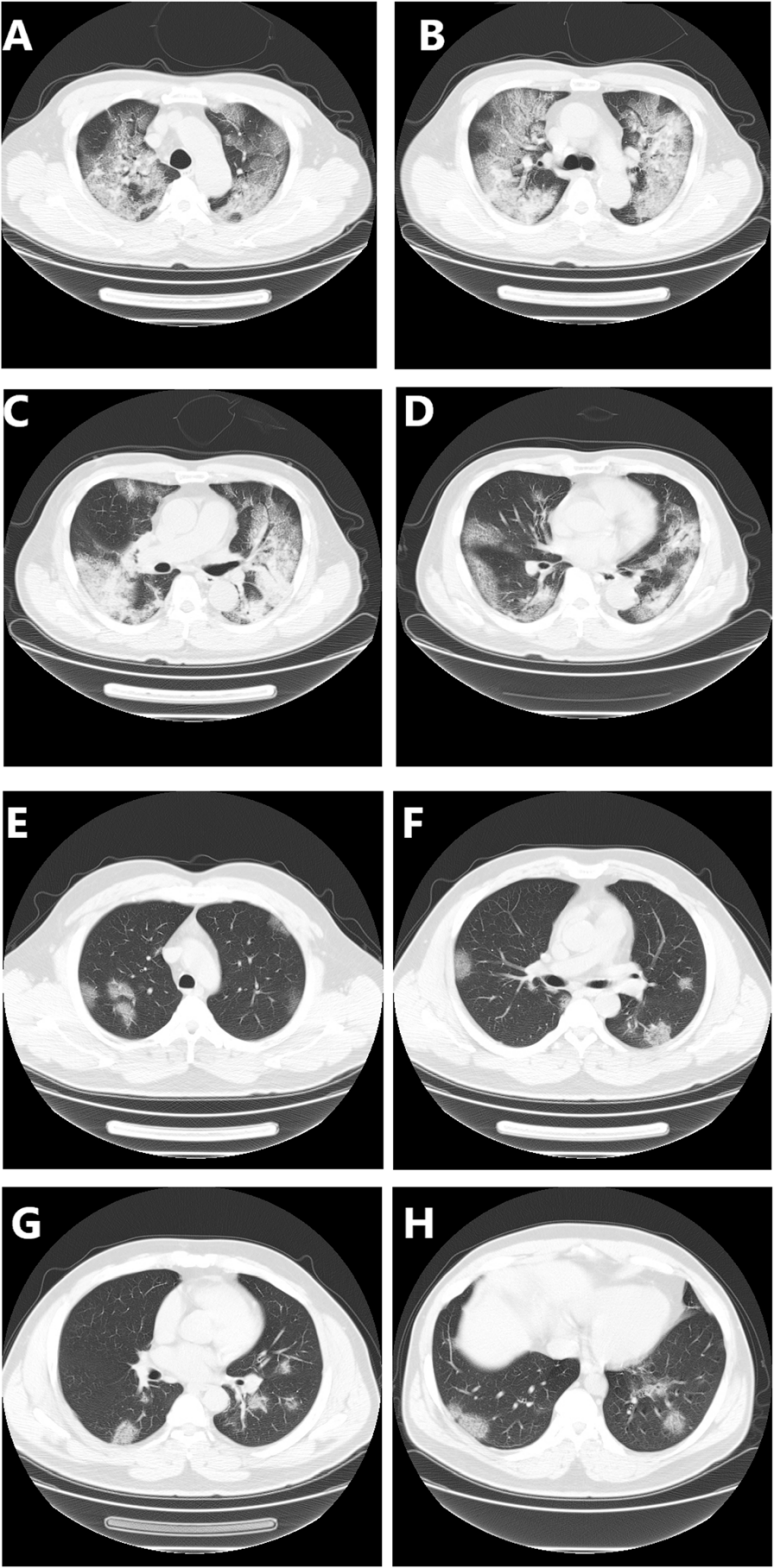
CT results of COVID-19 pneumonia patients. (a-d) CT results of severe patient;(e-g) CT results of general patient
